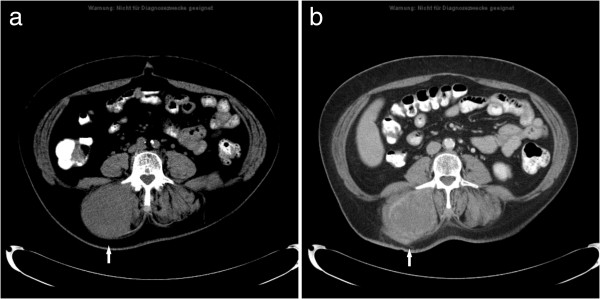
MM type III in a patient with known metastatic soft tissue sarcoma. CT images before (a) and after administration of contrast medium (b) documenting a massive hypodense enlargement of the right paravertebral musculature with inhomogeneous enhancement (arrow).
